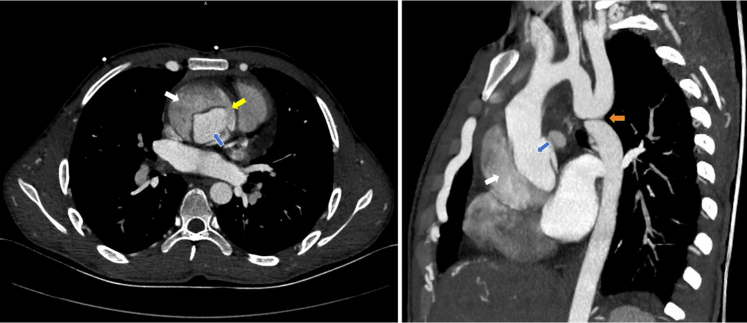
Preoperative computed tomography angiography demonstrating acute type A aortic dissection and interrupted aortic arch. Axial view: The *yellow arrow* shows the intimal tear in the ascending aorta; the *blue arrow* identifies the true lumen, and the *white arrow* indicates the false lumen. Sagittal view: The *blue* and *white arrows* correspond to the true and false lumens, respectively. The *orange arrow* shows the site of aortic interruption distal to the left subclavian artery.
